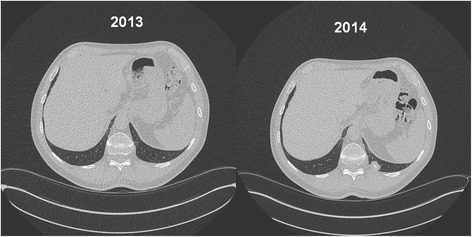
CT-scans of the thorax (*left*: 2013, *right*: 2014) showing identical levels of the costophrenic sinus, with a newly emerged large, smooth nodule paravertebral attached to the *left* chest wall (dimensions 21 × 13 mm, central calcification, no contrast material)
